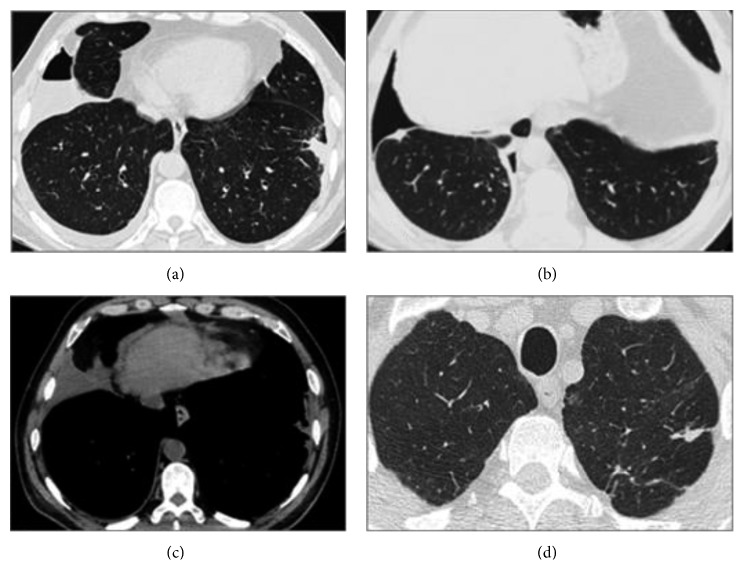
Computed tomography scan of the chest showing on the right lung: (a) hydropneumothorax, (b) basal pleural nodule, and (c) pleural thickening; on the left lung: (a) basal pleural residual changes and (d) upper lobe residual parenchymal changes consistent with past tuberculosis.
